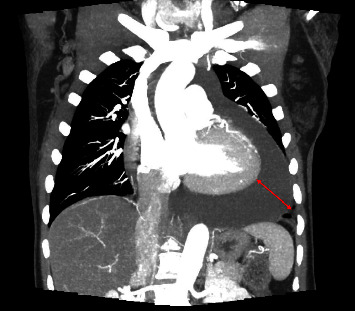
Coronal view of chest CT with angiography obtained due to concern for aortic dissection shows large pericardial effusion (red line) without aortic dissection, pericardial or pleural thickening, or pericardial or pleural masses. Bilateral axillary lymph nodes with normal reniform morphology were present and were thought to be reactive in the setting of large pericardial effusion.
